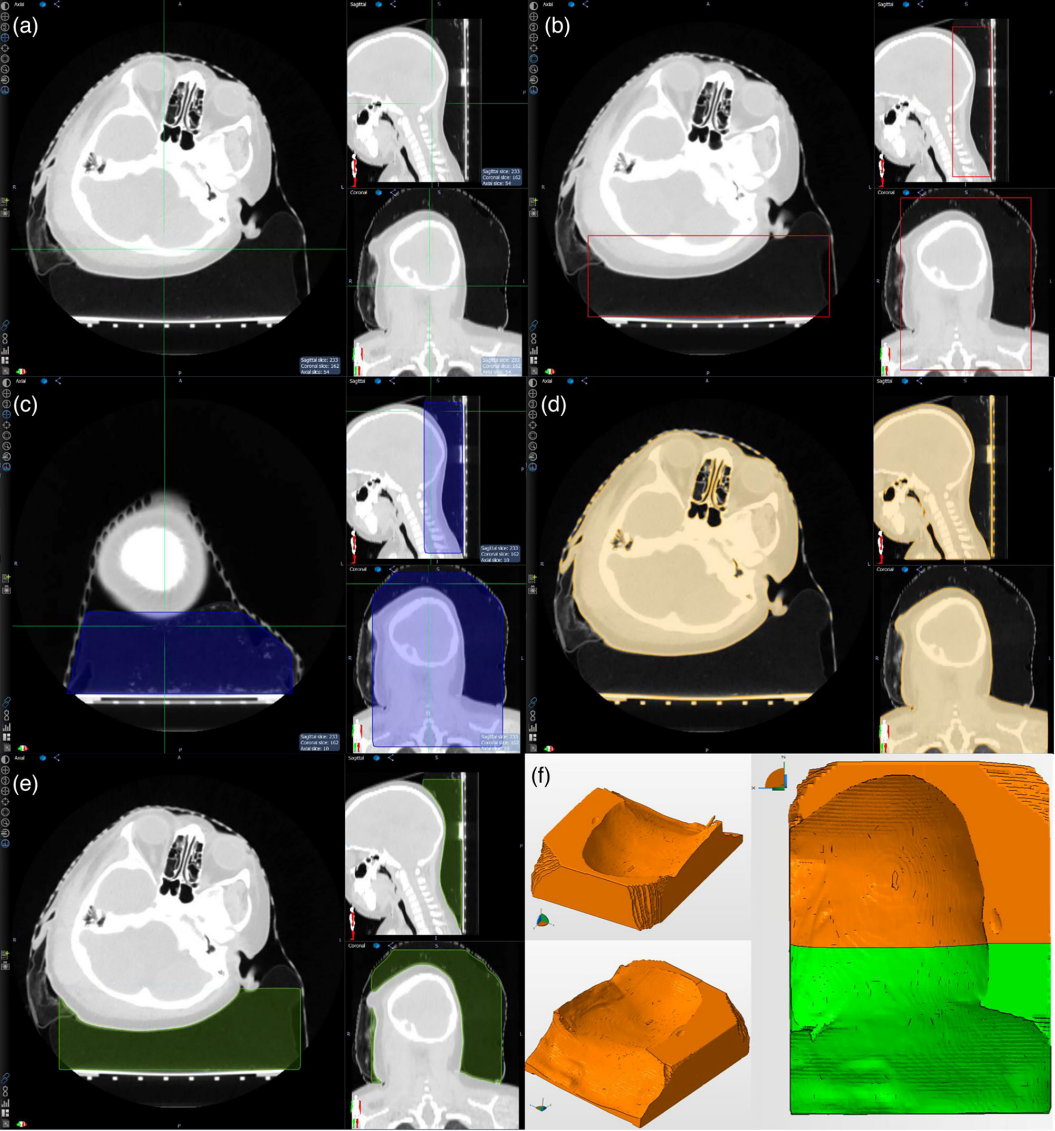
Generation of 3D model for head rest used in a brain treatment shown as follows: (a) planning CT with initial head rest visible, (b) cubic structure that encompasses roughly the region of the head, neck, and shoulders as the original head rest, (c) manual modification of the cubic structure to avoid overlap with the thermoplastic mask, (d) external body image, (e) new structure resulting from subtracting the external contour from the modified cubic structure, and (f) final 3D model is shown in three views, on the right orange (“Part 1″) and green (“Part 2″) regions indicate how the headrest was printed in two parts.
